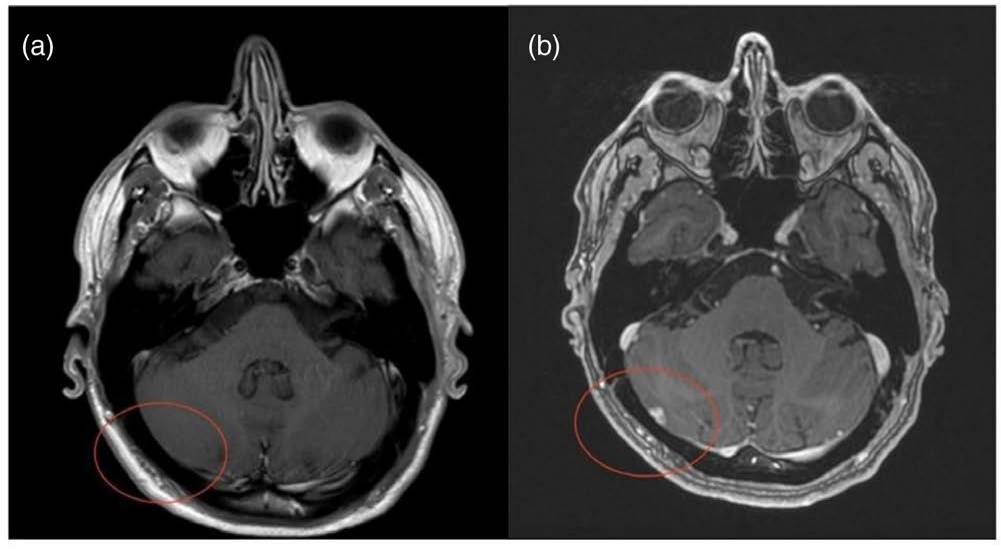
Comparison brain MRI between April 2022 (a) and June 2022 (b) when the cerebellar lesion appeared, after 17 months from the beginning of treatment with lorlatinib.
